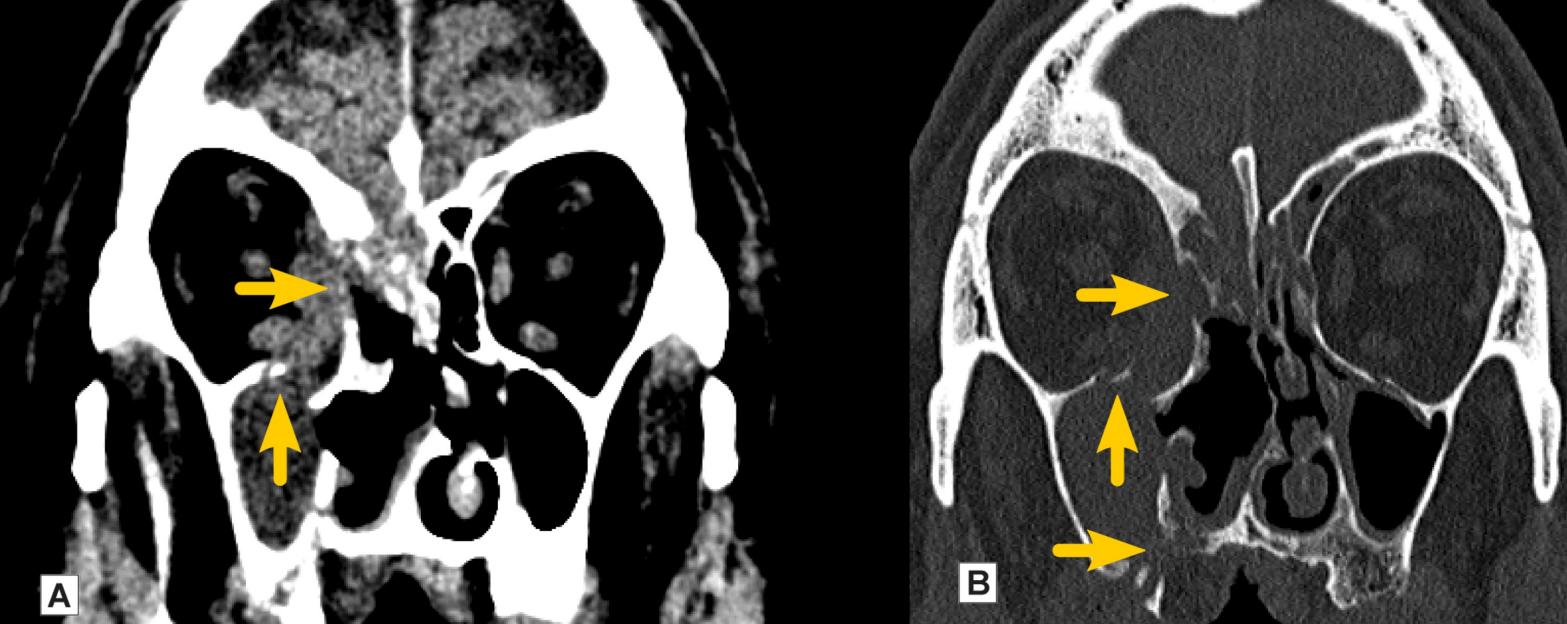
Native CT findings (Case 3) Native CT (A) and native CT bone window (B): filled with liquid density content of the right maxillary sinus with osteolysis of the right maxillary sinus's superior wall (arrow), the right orbit's medial wall (arrow), and oroantral fistula (arrow)
